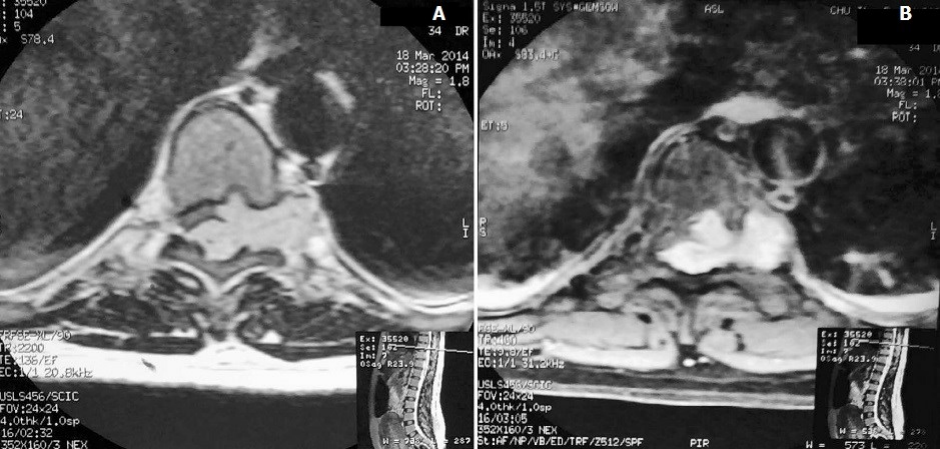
Transverse unenhanced: A) and contrast-enhanced; B) T1-weighted MR images show an epidural mass with homogenous intense enhancement, extending into and widening the left inter-vertebral foramen with a typical dumbell-shaped morphology and a bone erosion of vertebral body
